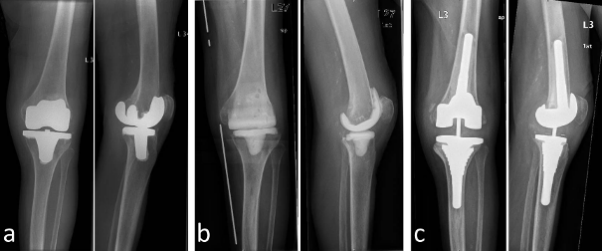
a) X-ray showing TKR with no signs of loosening, yet clinical signs of infection. b) Explantation of the components of TKR and dynamic cement spacer in situ. c) Reimplantation with a semi-constraint TKR.
